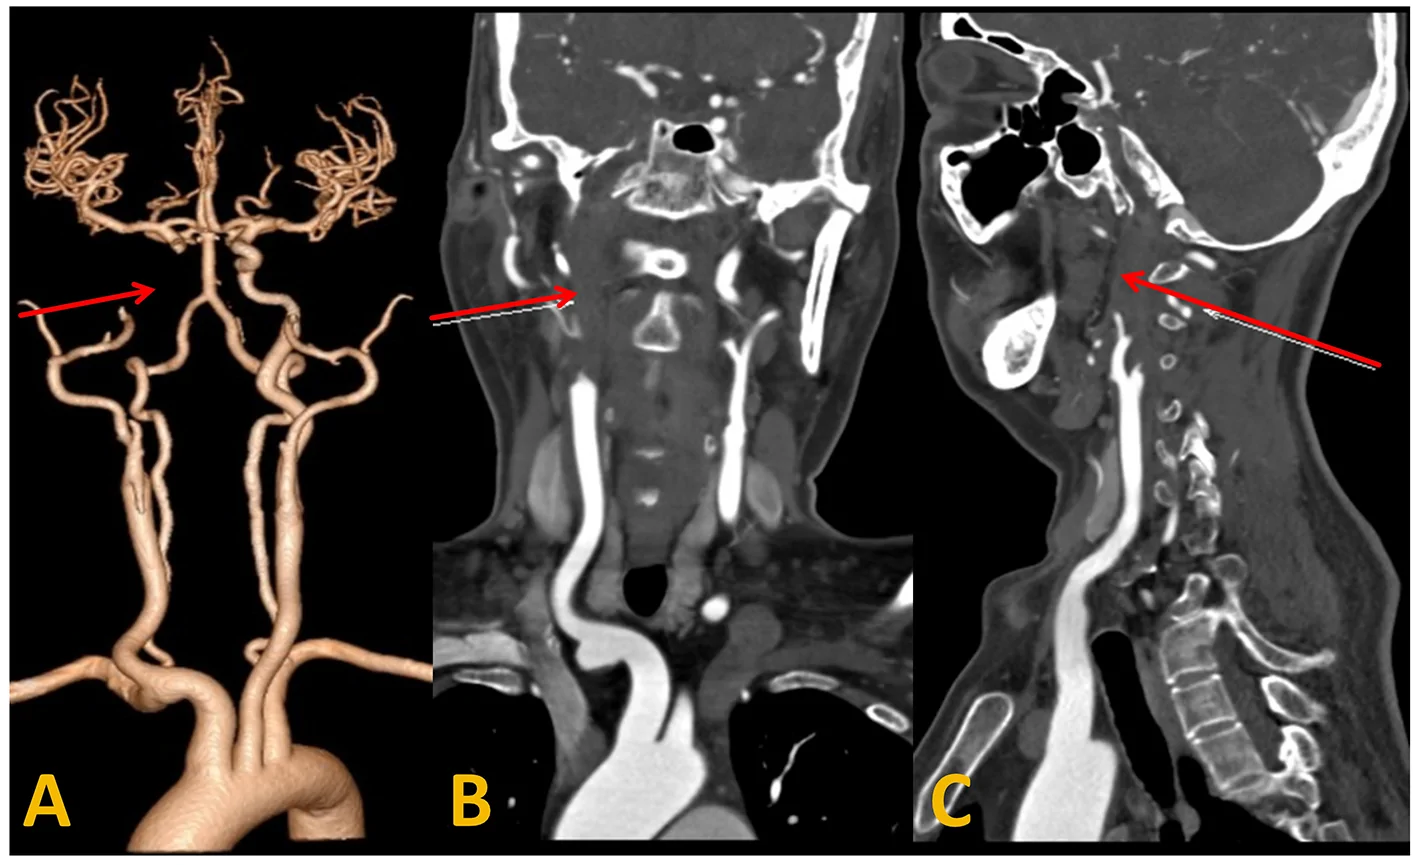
CTA reassessment conducted one month after patient admission. (A) 3D-CTA; (B) Coronal 2D-CTA; (C) Sagittal 2D-CTA. CTA demonstrates extensive occlusion of the right internal carotid artery, complete resolution of the aneurysm, patency of the anterior communicating artery, and satisfactory blood flow to the right middle cerebral artery and anterior cerebral artery.
